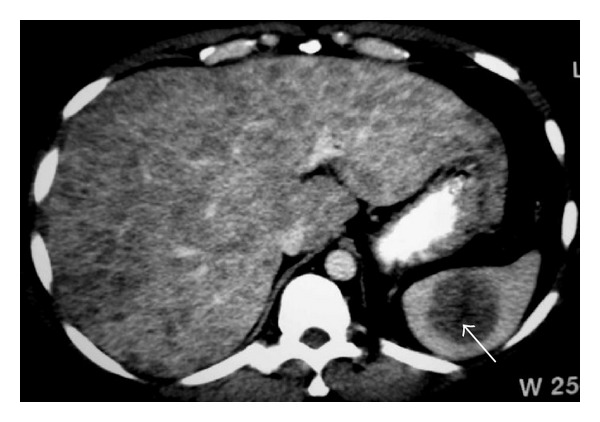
NHL. Axial contrast-enhanced CT image reveals multiple small low-attenuation nodules in the liver. Note also hypodense mass involving the spleen (arrow).
